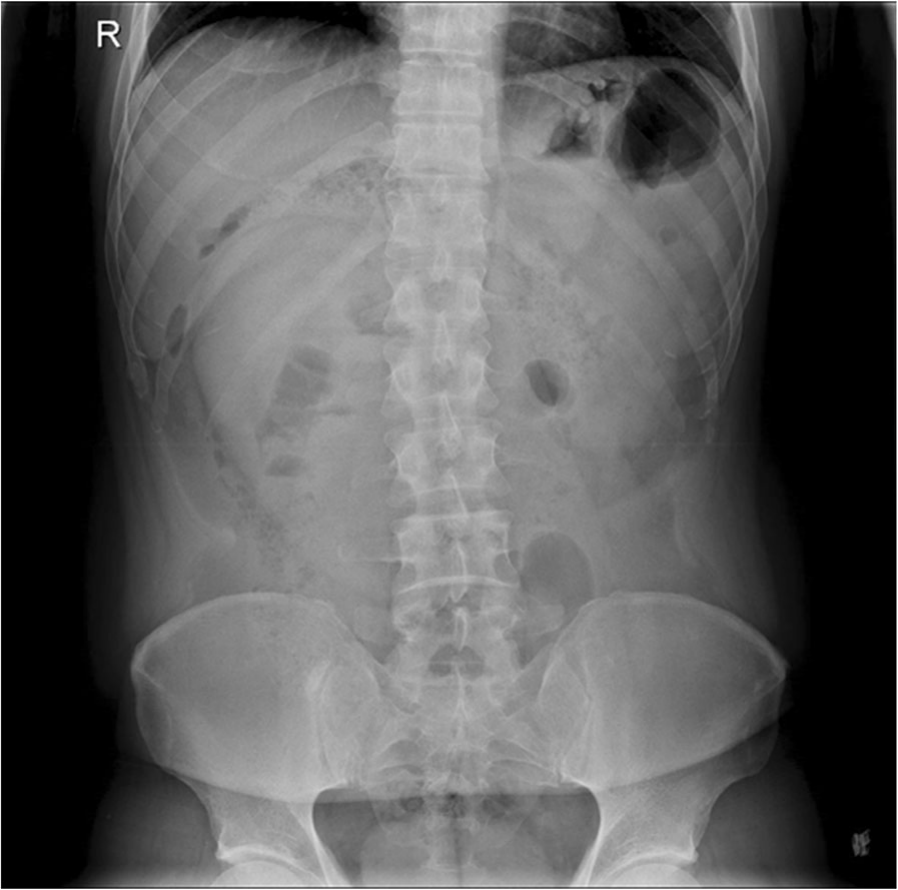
Abdominal X-ray showed a mass in the right upper quadrant of abdomen
